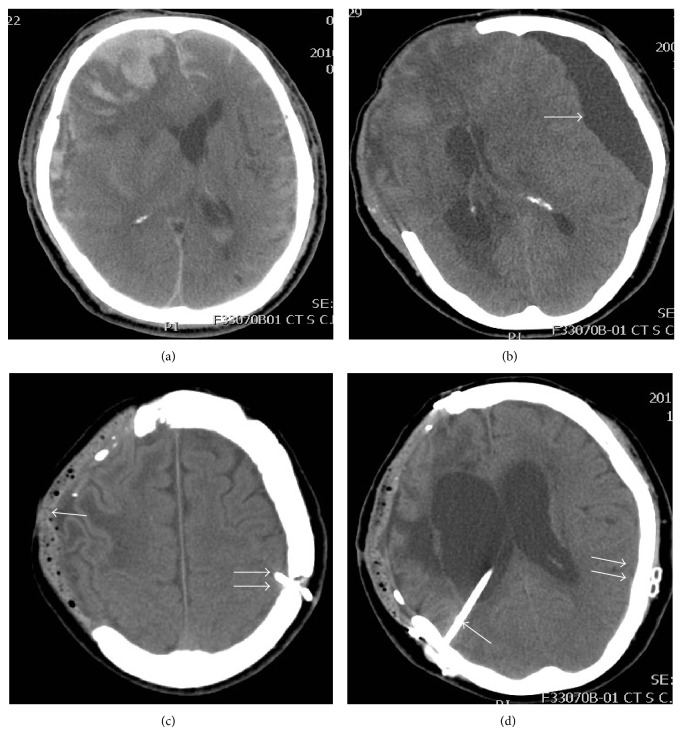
Representative pre- and postoperative CT images of case 1. (a) CT scan of case 1, revealing acute subdural hematoma, contusion hemorrhage, and subarachnoid hemorrhage in the right frontotemporal region, with significant mass effect. Wide craniectomy with the removal of hematoma was indicated. (b) A later follow-up CT scan revealed SDG contralateral to DC (arrow), with significant mass effect. (c) Simultaneous cranioplasty (single arrow) and contralateral SP shunting (double arrow) were performed and postoperative CT scan revealed completely resolved SDG and reversed midline shift. (d) A later follow-up CT scan revealed hydrocephalus and VP shunt (single arrow) following SP shunt (double arrow) insertion earlier to eliminate both hydrocephalus and contralateral SDG, respectively.
